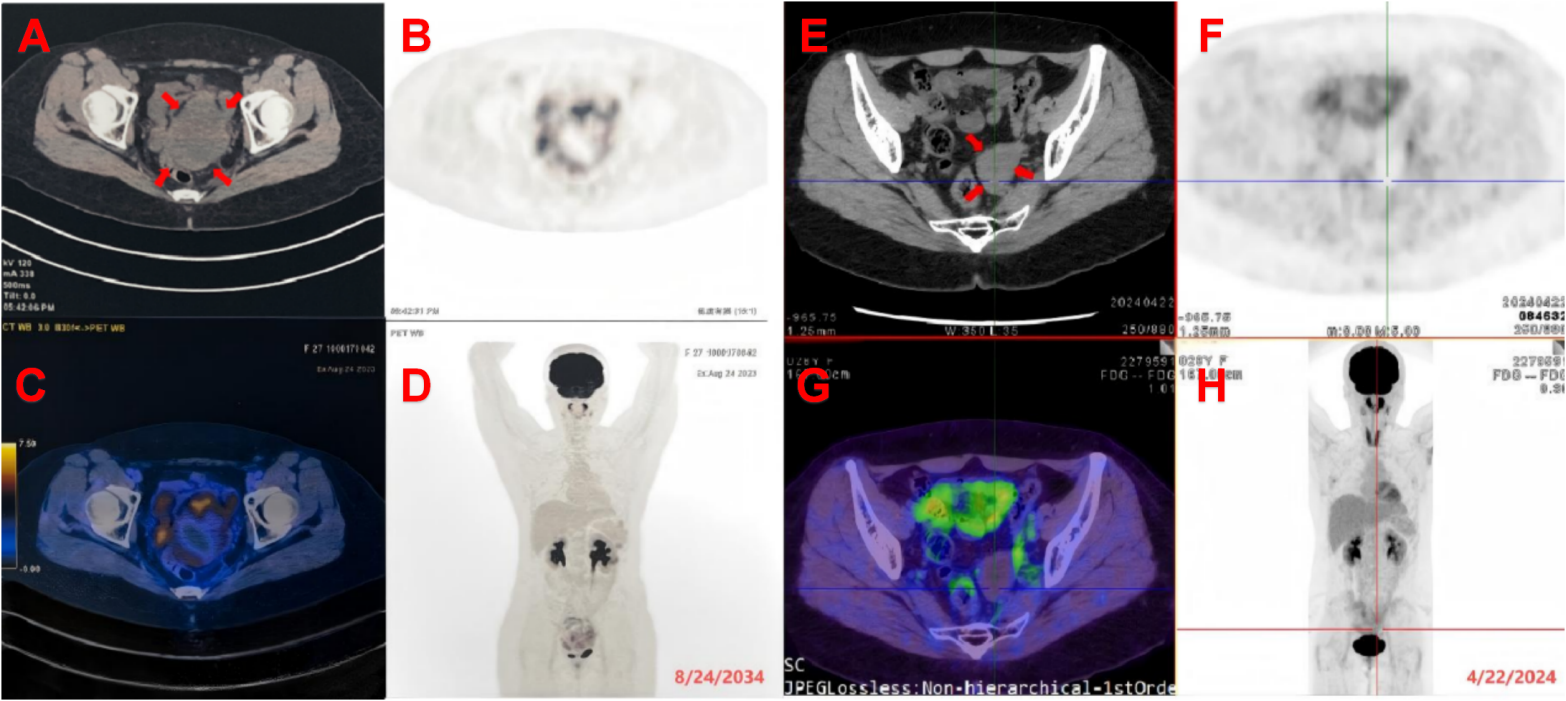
(A–D) Pre-treatment PET/CT: A pelvic soft tissue mass demonstrates heterogeneously intense radiotracer uptake with a maximum standardized uptake value (SUVmax) of approximately 7.0. The lesion measures approximately 8.7 × 8.3 × 9.0 cm, showing internal necrotic components. The mass invades the vaginal stump and exhibits ill-defined borders with the rectum and the pelvic segment of the left ureter. (E–H) Post-treatment PET/CT after 6 courses of chemotherapy and 2 sessions of HIFU therapy.A hypodense lesion is noted in the left pelvis, measuring approximately 4.7 cm × 3.1 cm. It demonstrates ill-defined borders with the vaginal stump and has an SUVmax of 2.8.
